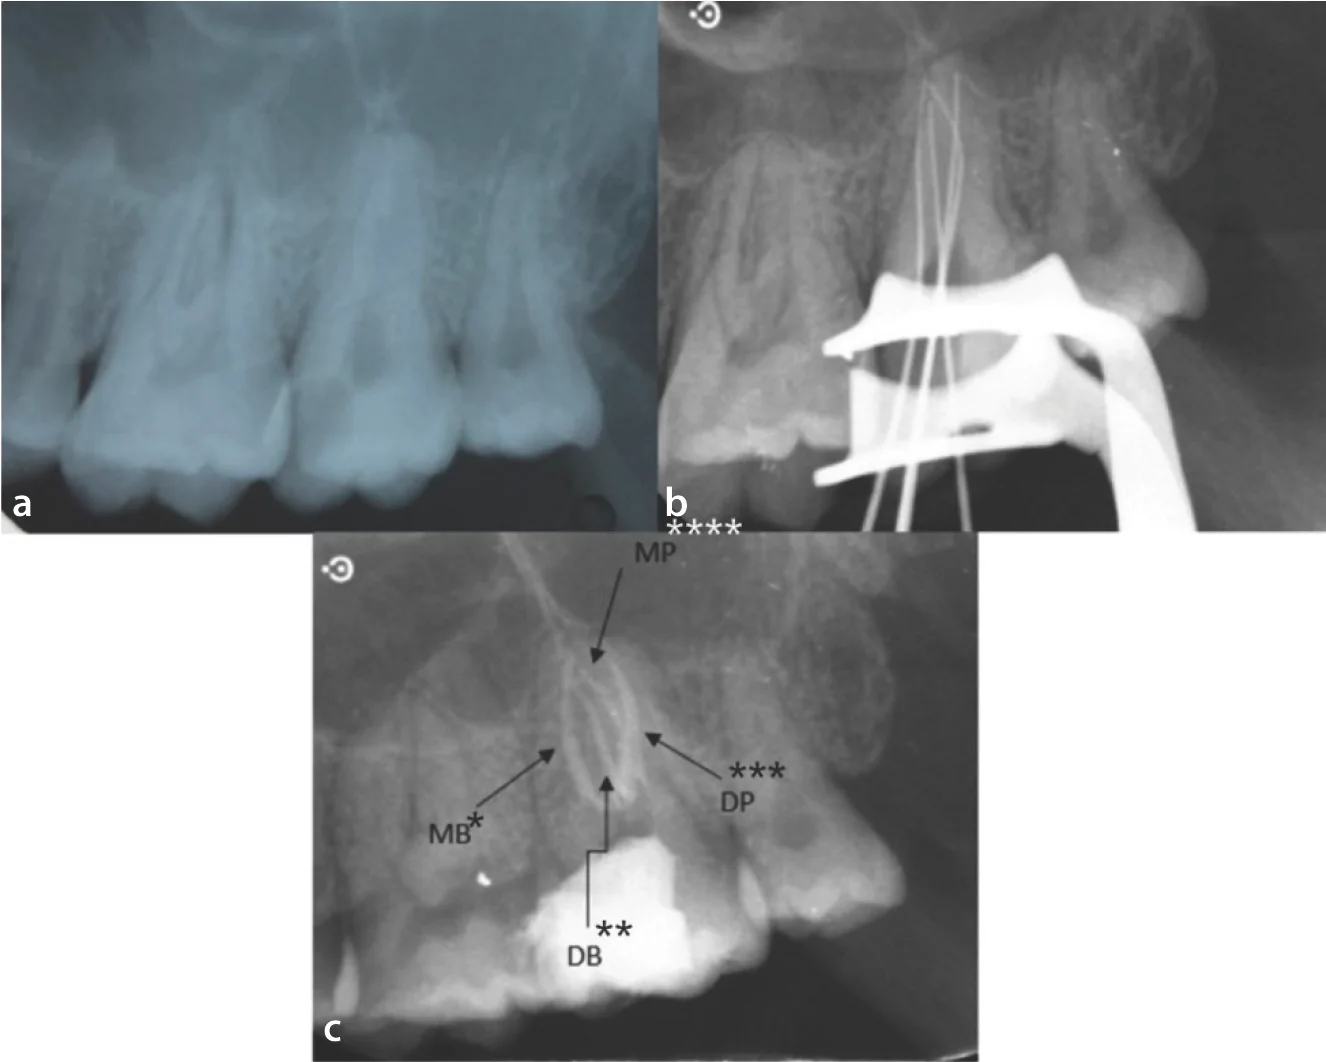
a: Pre operative radiography, b: Initial files for working length, c: Post operative radiography showing 4 canals of this case as (* MB: mesiobuccal, DB: distobuccal, *DP: distopalatal, MP: mesiopalatal)
